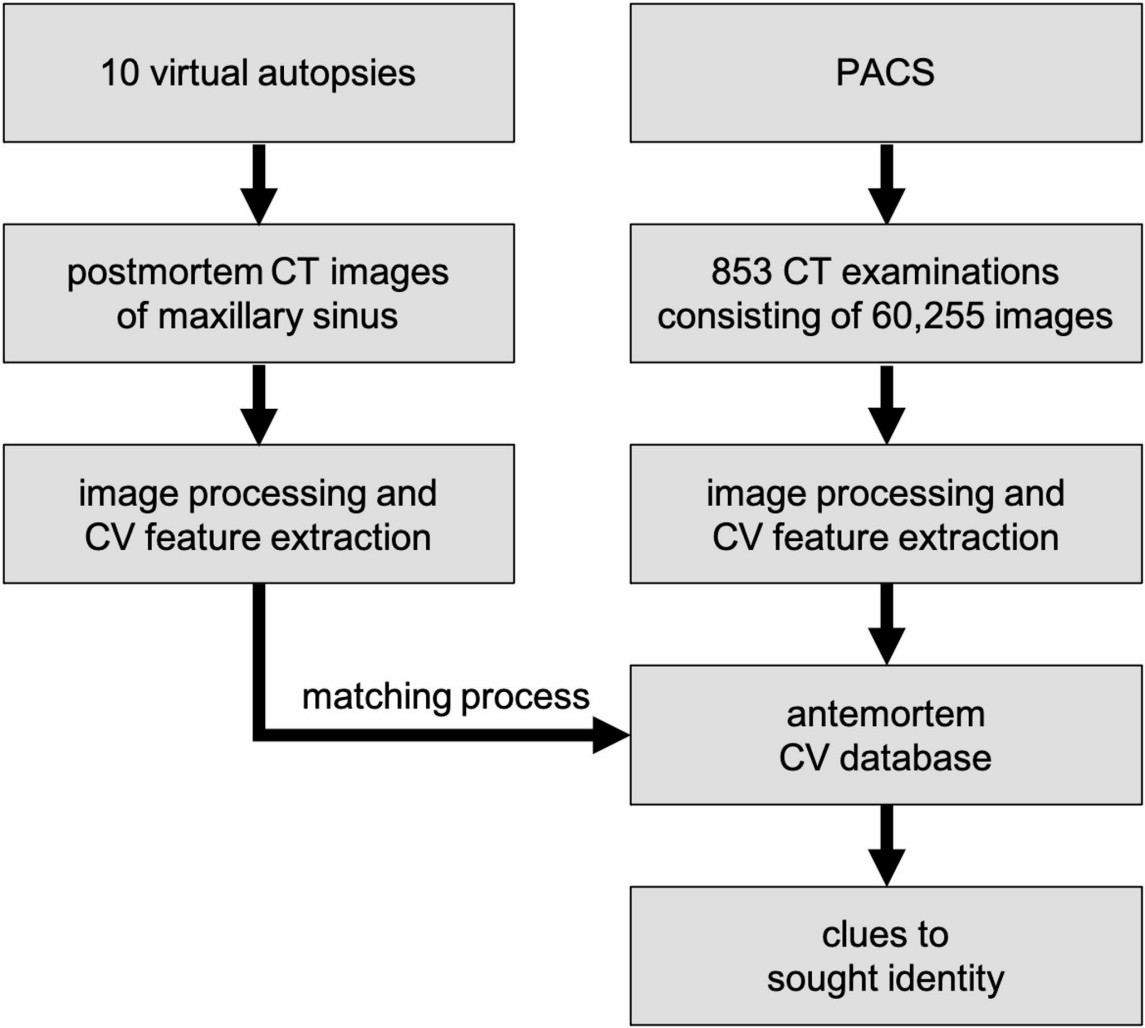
For 10 virtual autopsies, CT images of the maxillary sinus were selected for each case. CV features were extracted and compared with antemortem CV data to identify the 10 sought individuals. The antemortem CV database included CT slices from 853 examinations, exported from the picture archiving and communication system (PACS)
